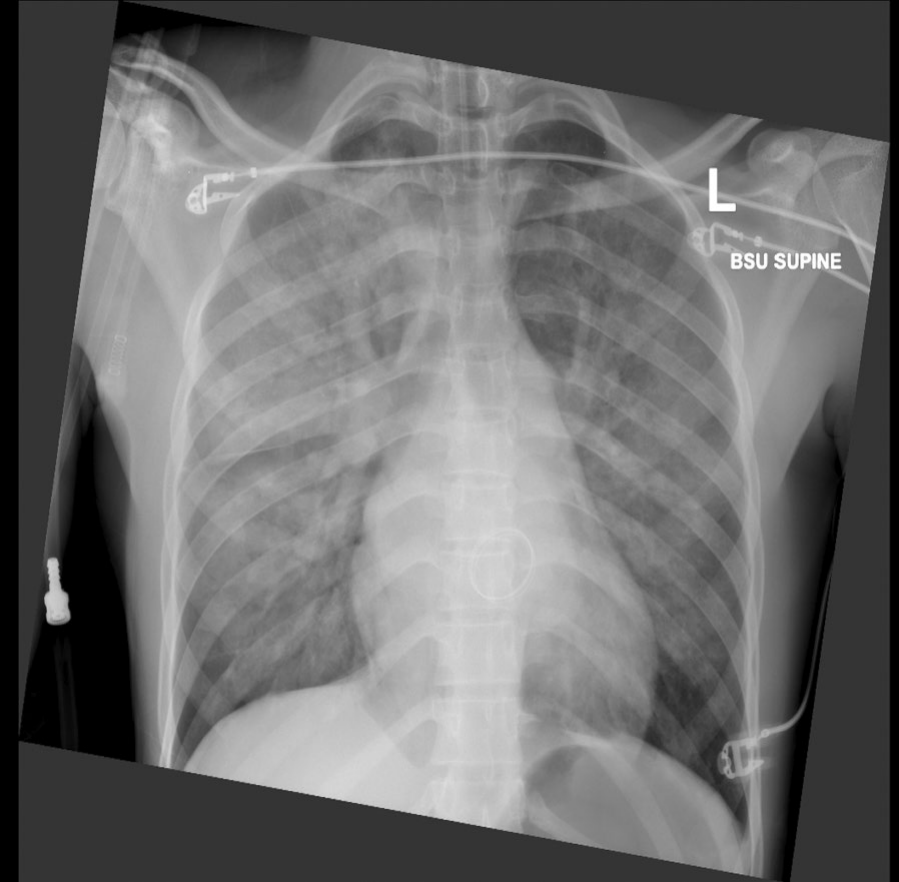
Chest radiograph showing pulmonary oedema
